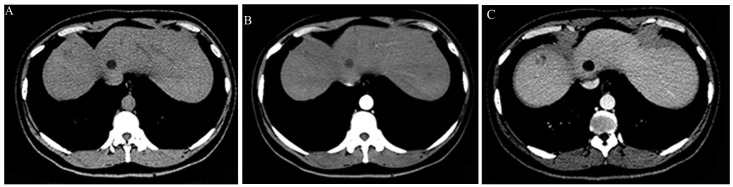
Computed tomography scans revealing (A) a 1.3-cm well-defined tumor in segment eight of the liver. The tumor presents (B) punctate enhancement in the early phase while (C) the enhancement becomes stronger in the delayed phase.
